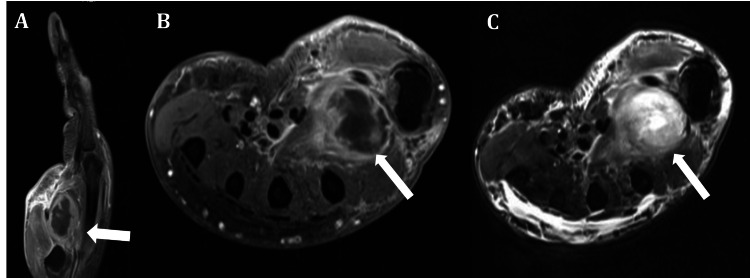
Magnetic resonance imaging. A. T1W post-contrast sagittal view, B. T1W post-contrast transverse view, and C. T2W post-contrast transverse view, depicting abscess at the thenar eminence of the right hand (arrows).
